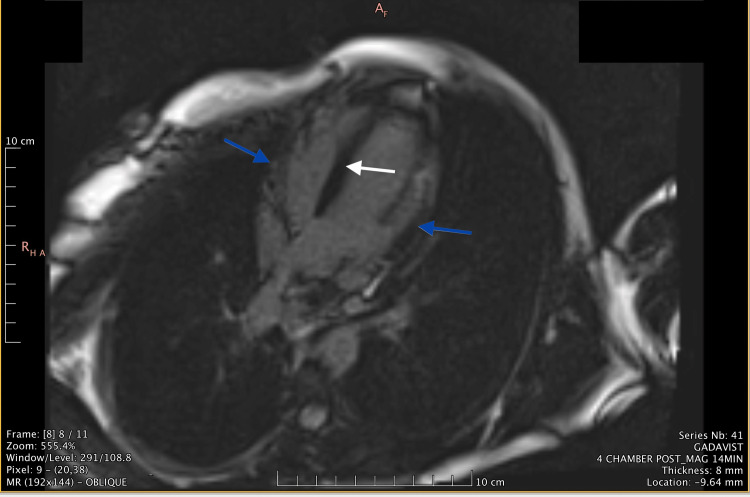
Oblique MRI image demonstrating late myocardial enhancement in a non-ischemic pattern within the septum and lateral wall characteristic of myonecrosis or scar Blue arrows indicate the areas of late myocardial enhancement in the lateral walls and white arrow indicates the areas of late myocardial enhancement in the septum MRI: magnetic resonance imaging
